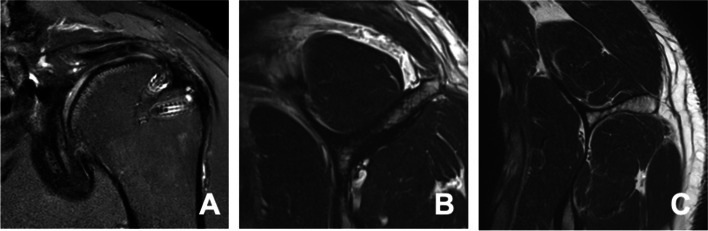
A Postoperative coronal MRI showing intact supraspinatus tendon fixed in double-row technique. B Preoperative parasagittal MRI of supraspinatus muscle showing good muscle quality slight atrophy due to retraction. C Postoperative parasagittal MRI of supraspinatus muscle showing no progression of muscle atrophy or fatty infiltration
